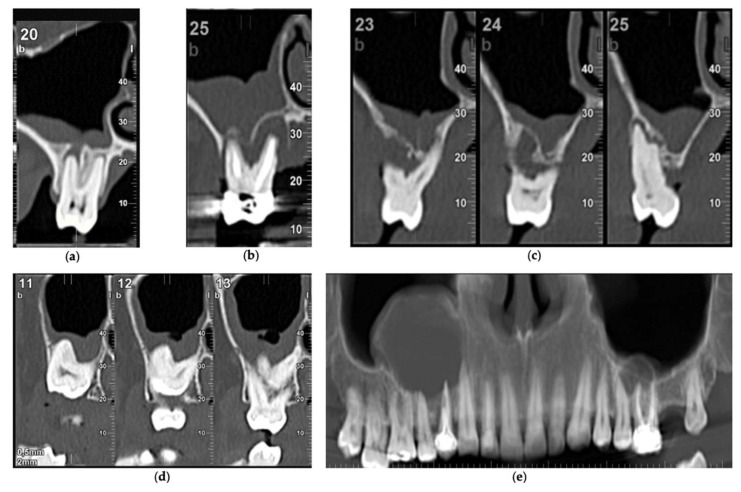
Dental factors triggering sinus thickening: (a) periapical pathology; (b) endodontic treatment; (c) periodontitis; (d) impacted third molar; (e) dental radicular cysts.
